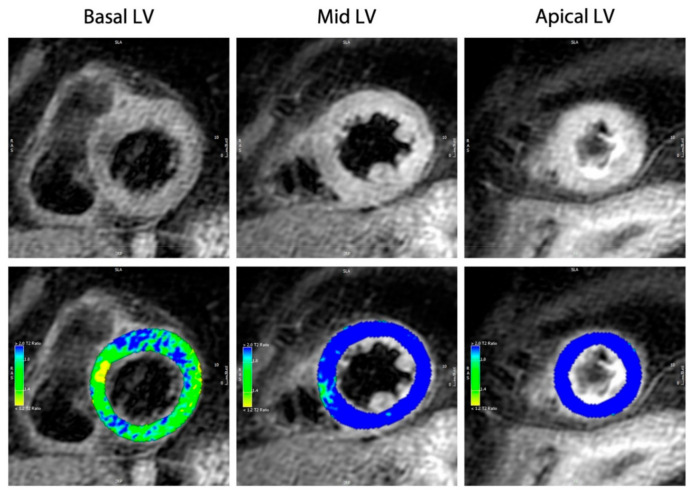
T2-weighted images (short-axis view) demonstrating normal signal intensity (SI) of the basal myocardium and global edema of the mid and apical myocardium. SI analysis (bottom row) of the T2-weighted images with color-coded display of relative SI normalized to skeletal muscle (blue indicates an SI ratio of myocardium to skeletal muscle of ≥1.9 or higher, thus indicating edema; green/yellow indicates a normal SI ratio of <1.9).
